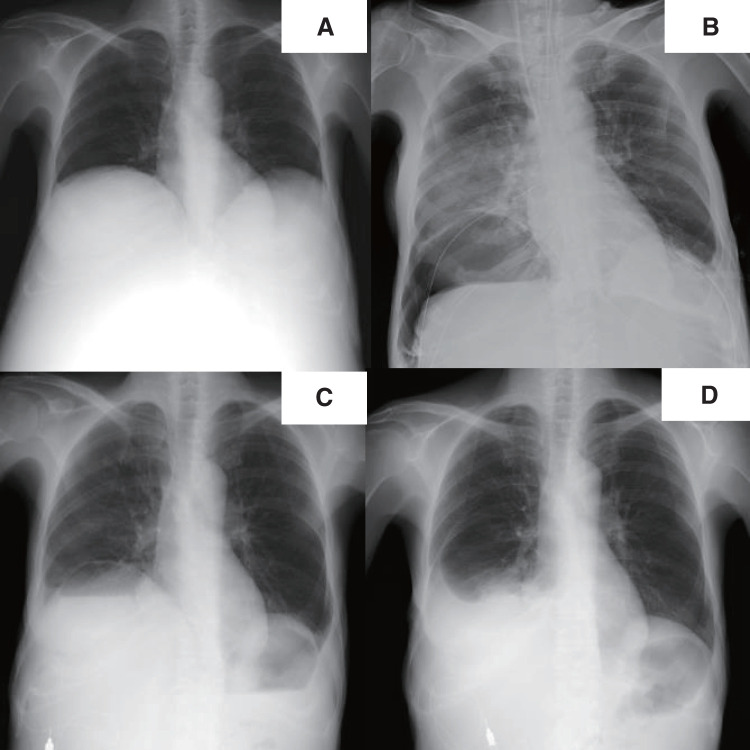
X-ray imaging. Before liver transplantation, the right diaphragm was significantly elevated (A). From the first day after transplantation, a cystic shadow was observed in the right lower lung field (B), and an air–fluid level was observed within the cyst before the pneumatocele operation (C). There was no cystic shadow on the chest X-ray image at the time of discharge (D).
